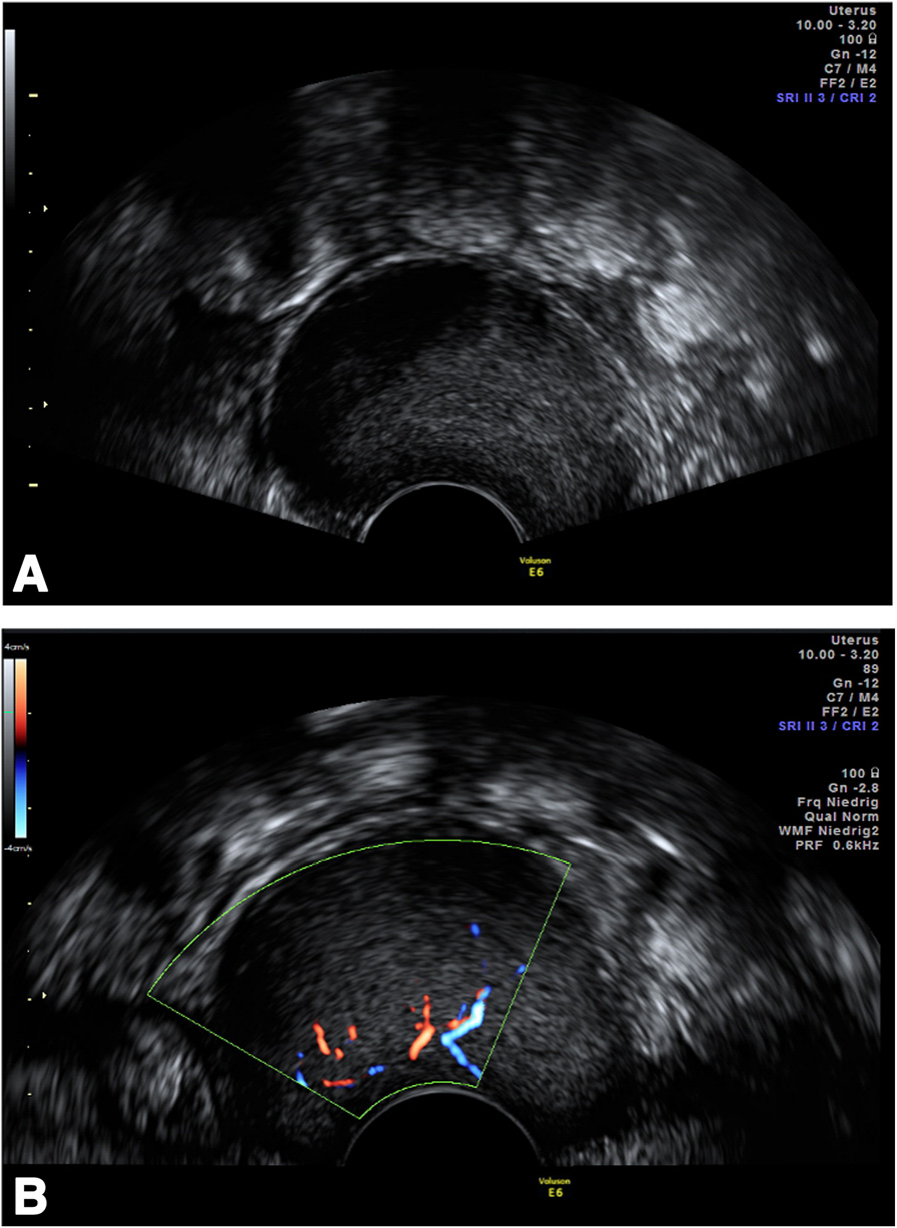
a Transvaginal ultrasound image shows a well-demarcated, homogeneous mass of medium echogenicity (sagittal view). Intralesional septations can be seen only in Additional file 1. b Color Doppler imaging reveals intralesional vascularization
